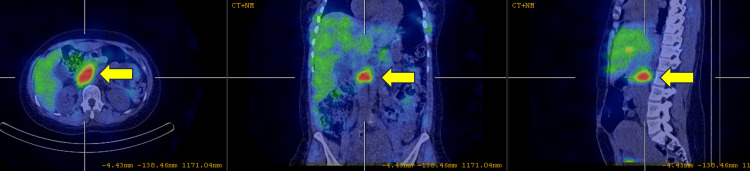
An iodine meta-iodobenzylguanidine (MIBG) scintigraphy scan confirming tracer uptake to a soft tissue mass at the level of the L2 vertebra.
